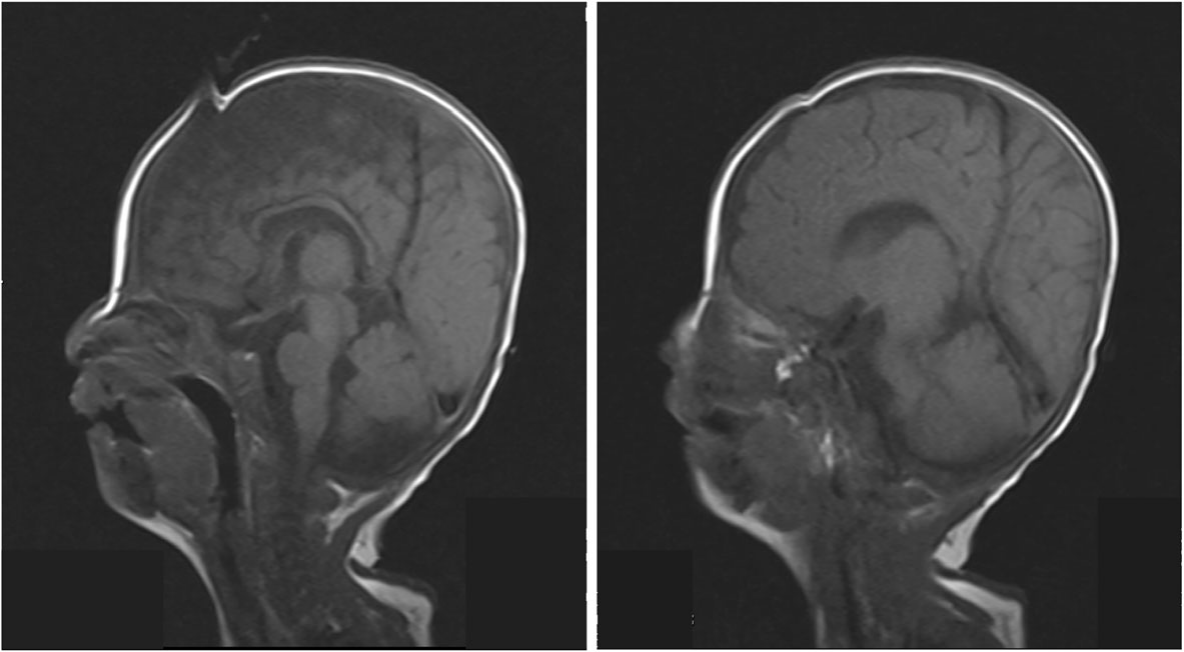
MRI image of head. Persistent falcine sinus and a thin corpus callosum confirmed by MRI
